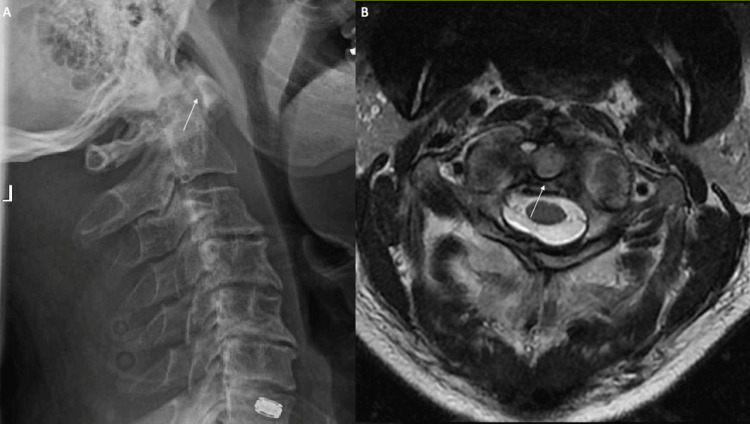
(A) Lateral cervical X-ray shows increased atlantodental interval of 5 mm, indicating instability (white arrow). (B) Axial T2 MRI demonstrated transverse ligament disruption (white arrow).
